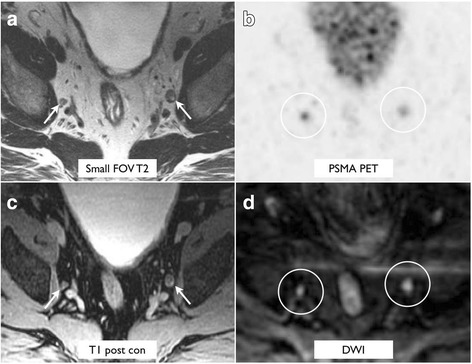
A 67-year-old man with biochemical recurrence (PSA of 8.0), who had bilateral pelvic side wall lymph nodes measuring 0.7 and 1.0 cm seen on T2-weighted imaging (a), PSMA PET (b), post-gadolinium T1-weighted imaging (c), and DWI (d)
